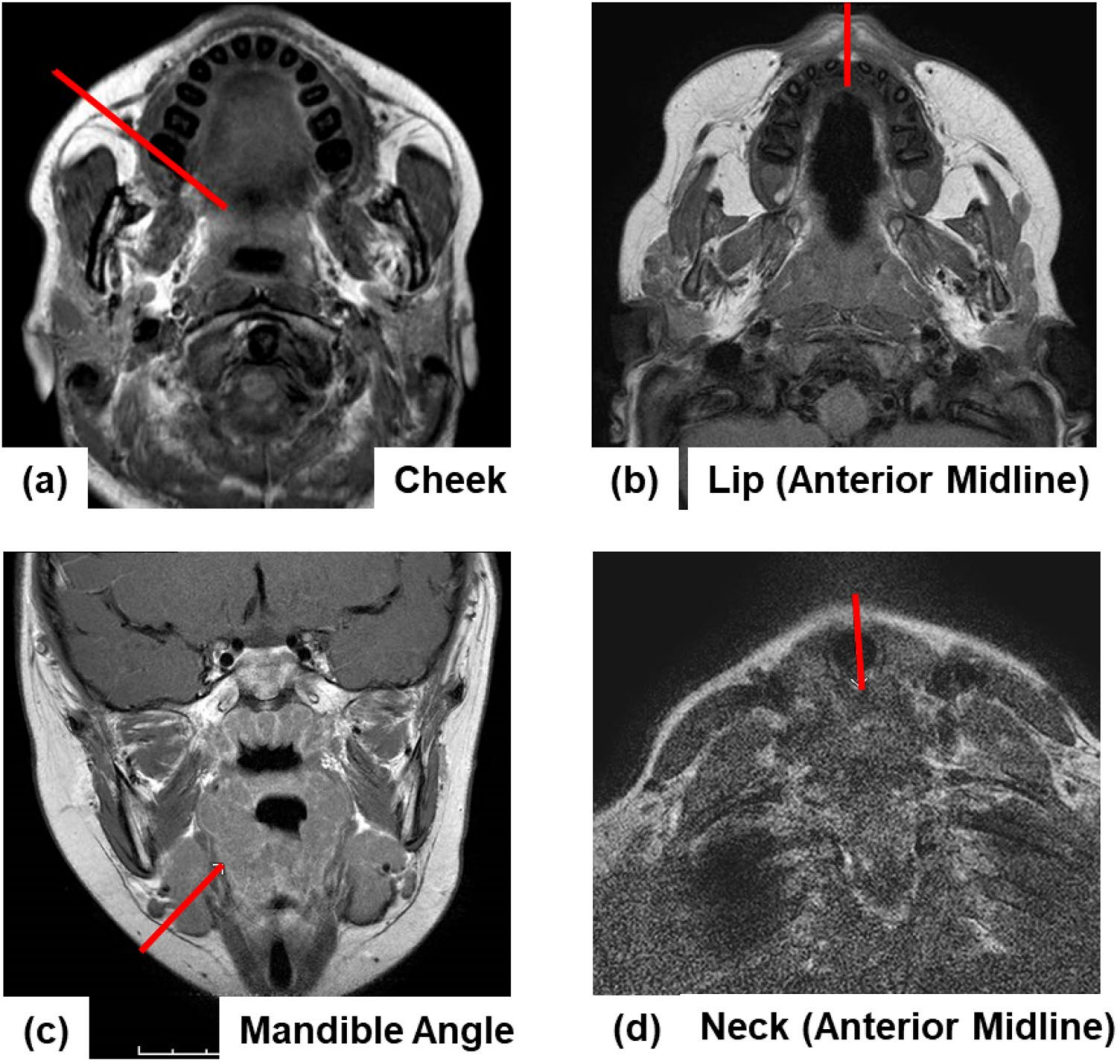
Example MR Images. Example MR images of cheek (a), lip (anterior midline) (b), mandible angle (c), and neck (anterior midline) (d) treatment sites. Tissue types and thicknesses were recorded along each trajectory indicated by the red line.
